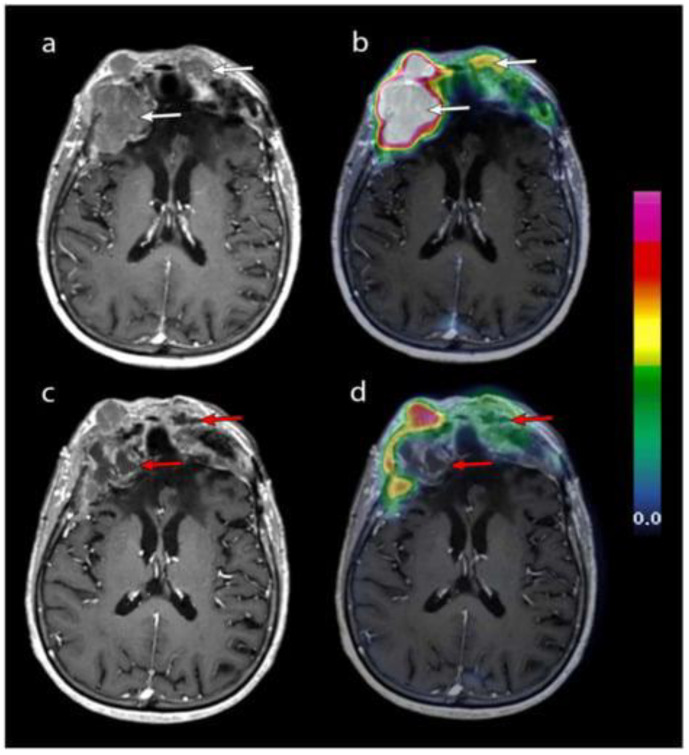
Contrast-enhanced T1-weighted axial MRI after the second (a) and fourth (c) cycles of PRRT, merged with 68Ga-DOTATOC PET (panels (b) and (d), respectively). Two frontal lesions (white arrows) exhibiting high 68Ga-DOTATOC uptake underwent necrosis after four cycles (red arrows). However, the underlying cause of this necrosis remains uncertain, as it could result from a direct effect of PRRT or natural tumor progression. Reprinted from [8] under CC BY license.
